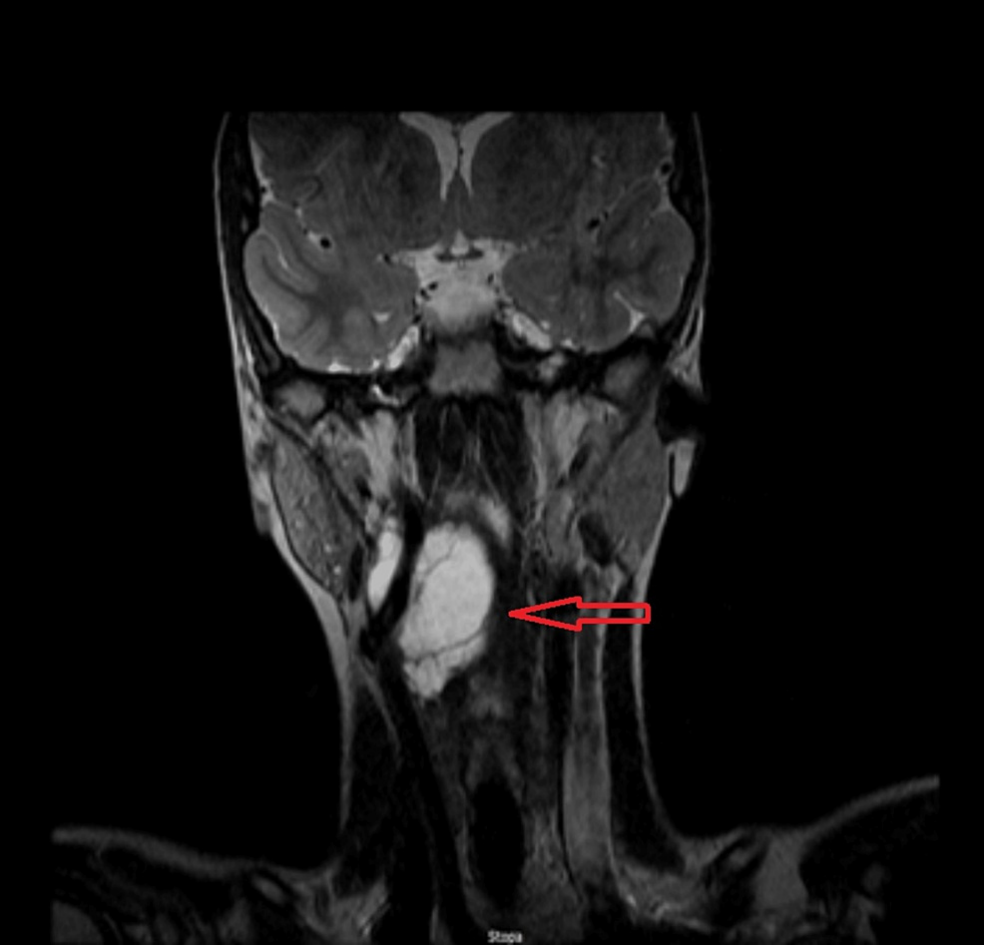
Magnetic resonance imaging, coronal T2-weighted images showing a retropharyngeal lesion extending to the right of the midline and covering the anterior part of the common carotid artery. Red arrow shows a lesion of interest.
